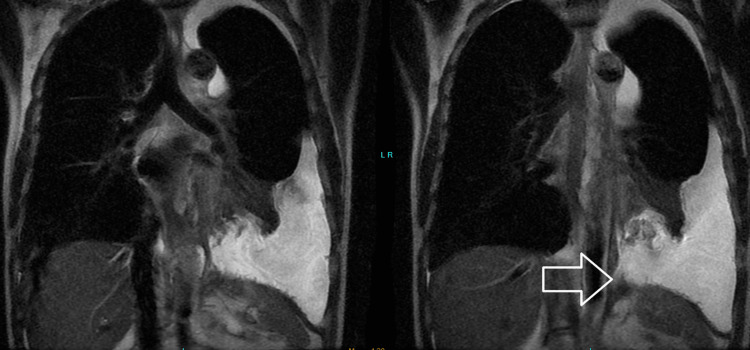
MRI of the chest demonstrating pancreaticopleural fistula (arrow pointing out the fistula tract).
